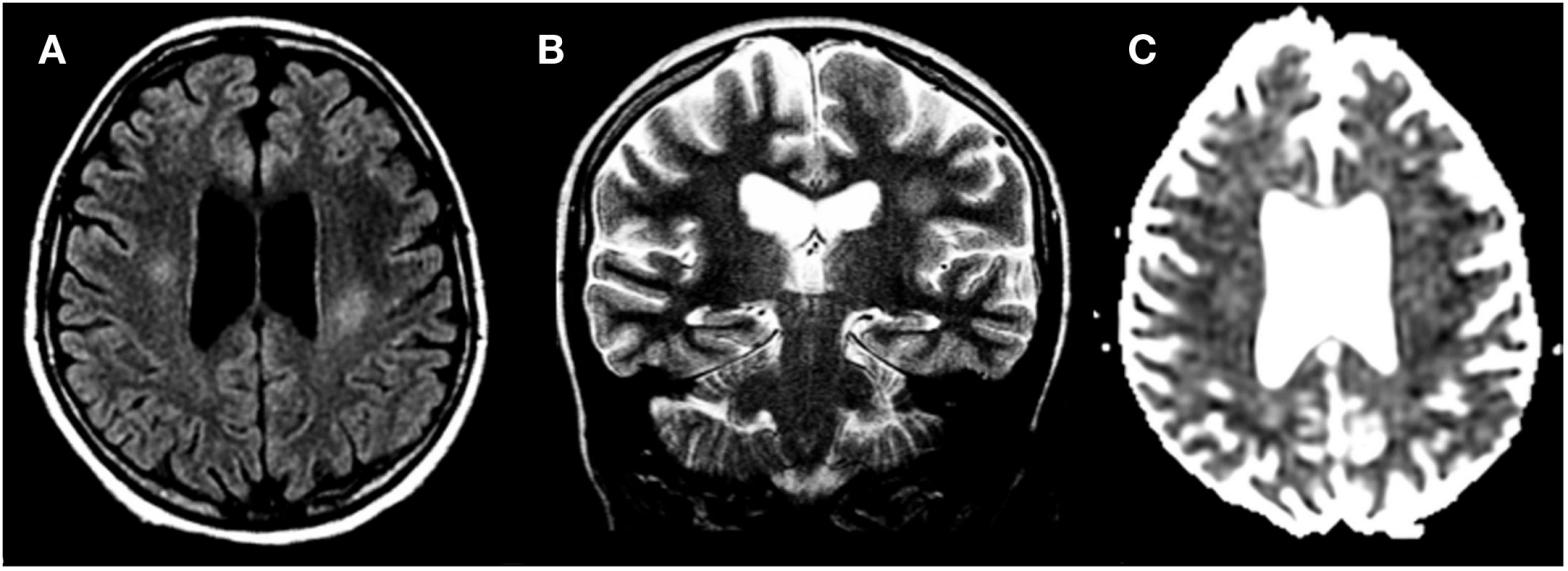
Methotrexate-induced leukoencephalopathy in a 13-year-old girl with acute lymphoblastic leukemia. (A,B) Axial FLAIR T2 and coronal T2-weighted images, respectively, reveal focal areas of hyperintensities within the deep cerebral white matter. (C) Diffusion study shows no cytotoxic edema.
